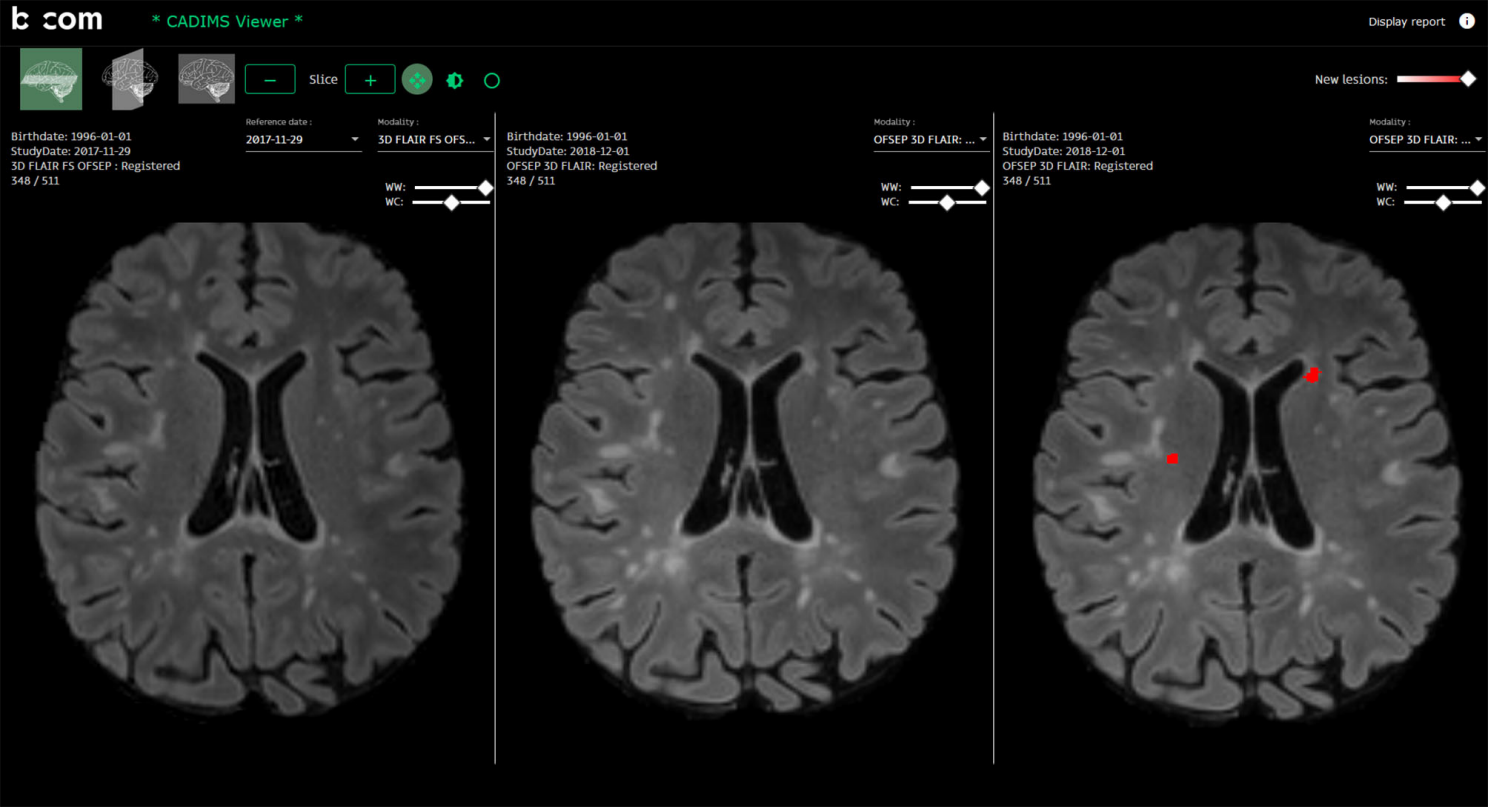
The CADIMS viewer: The CADIMS MRI viewer is usable from a standard web browser. It consists of three synchronized views displaying from left to right (i) the baseline scan, (ii) the follow-up scan and (iii) the follow-up scan with segmented new lesions highlighted in red.
